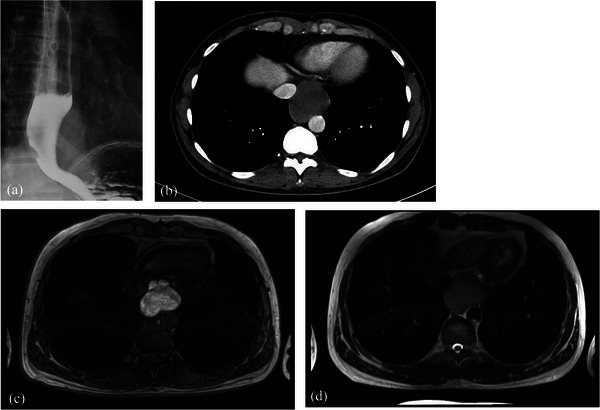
(a) Upper gastrointestinal radiography shows a lesion 50 mm in diameter on the left wall of the lower esophagus. (b) Contrast‐enhanced computed tomography shows a 52 × 44 mm homogeneous mass attached to the lower thoracic esophagus. (c) T1‐weighted magnetic resonance imaging reveals the mass to be multifocal with a high signal. (d) T2‐weighted magnetic resonance imaging reveals a slightly high signal for the mass.
